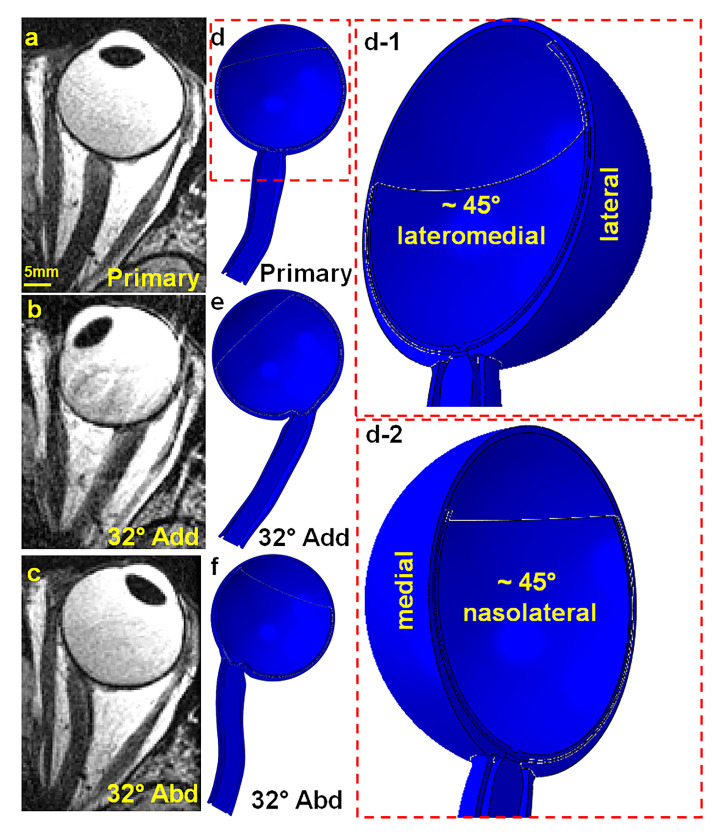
Axial MRI views of the left orbit: (a) primary gaze, (b) 32° adduction, and (c) 32° abduction. Hemisymmetric axial view generated by ABAQUS for (d) primary gaze (d-1) in ~45° lateromedial perspective and (d-2) ~45° nasolateral perspective, (e) 32° adduction, and (f) 32° abduction.
